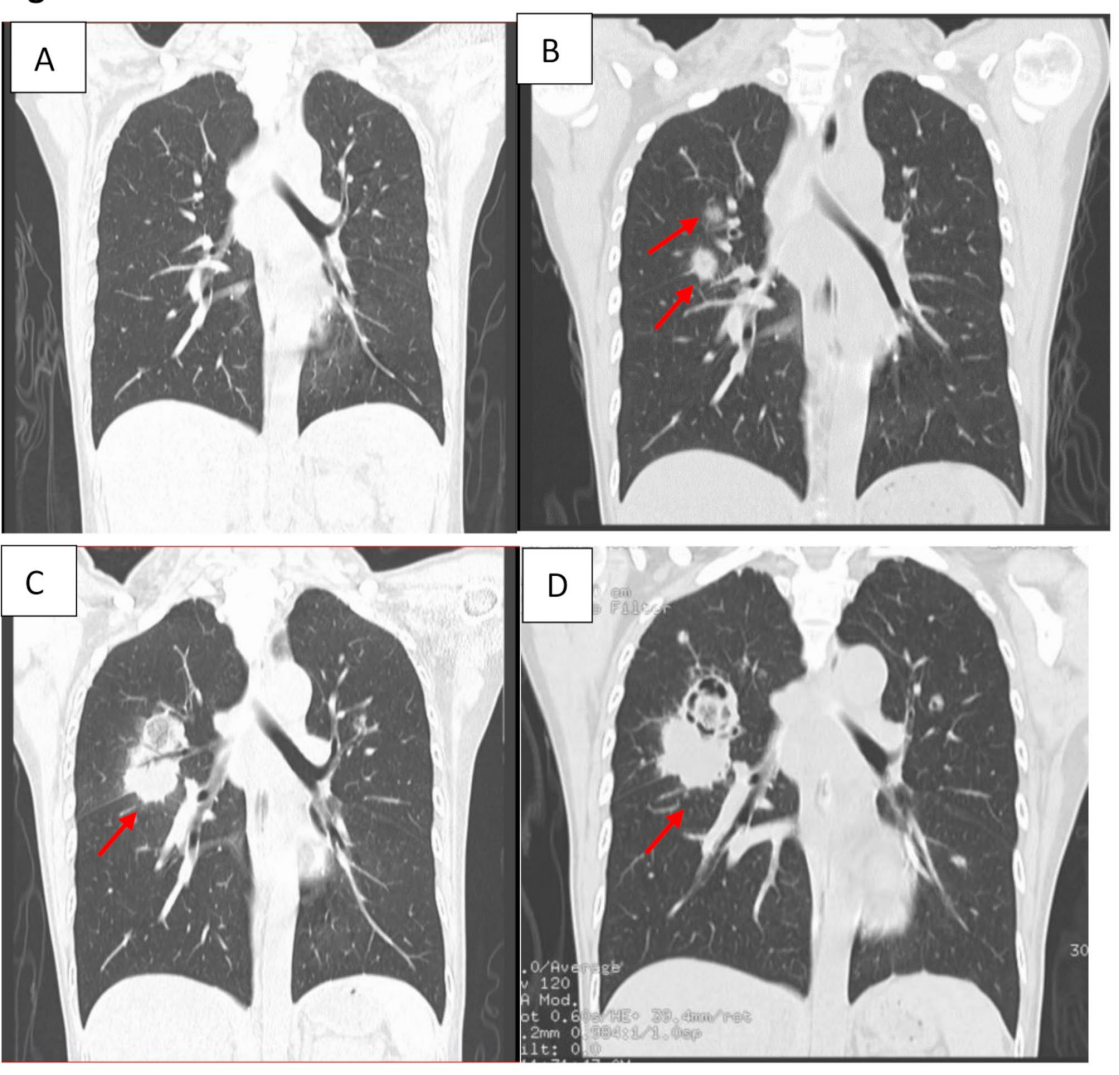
CT thorax (sagittal view) showing the progression of the lung lesions (arrows). A: No signs of pneumonia and pulmonary lesions on day 9; B: Two new pulmonary nodules (the biggest one is 1.9 × 1.5 cm) located in the right middle lobe surrounded by a halo of ground-glass opacity on day 21; C: Progression of the right middle lung mass (the biggest one is 3.5 × 5.2 cm) with cavitation on day 33; D: Progression of the right middle lung mass(3.6 × 5.2 cm) with cavitation on day 41
